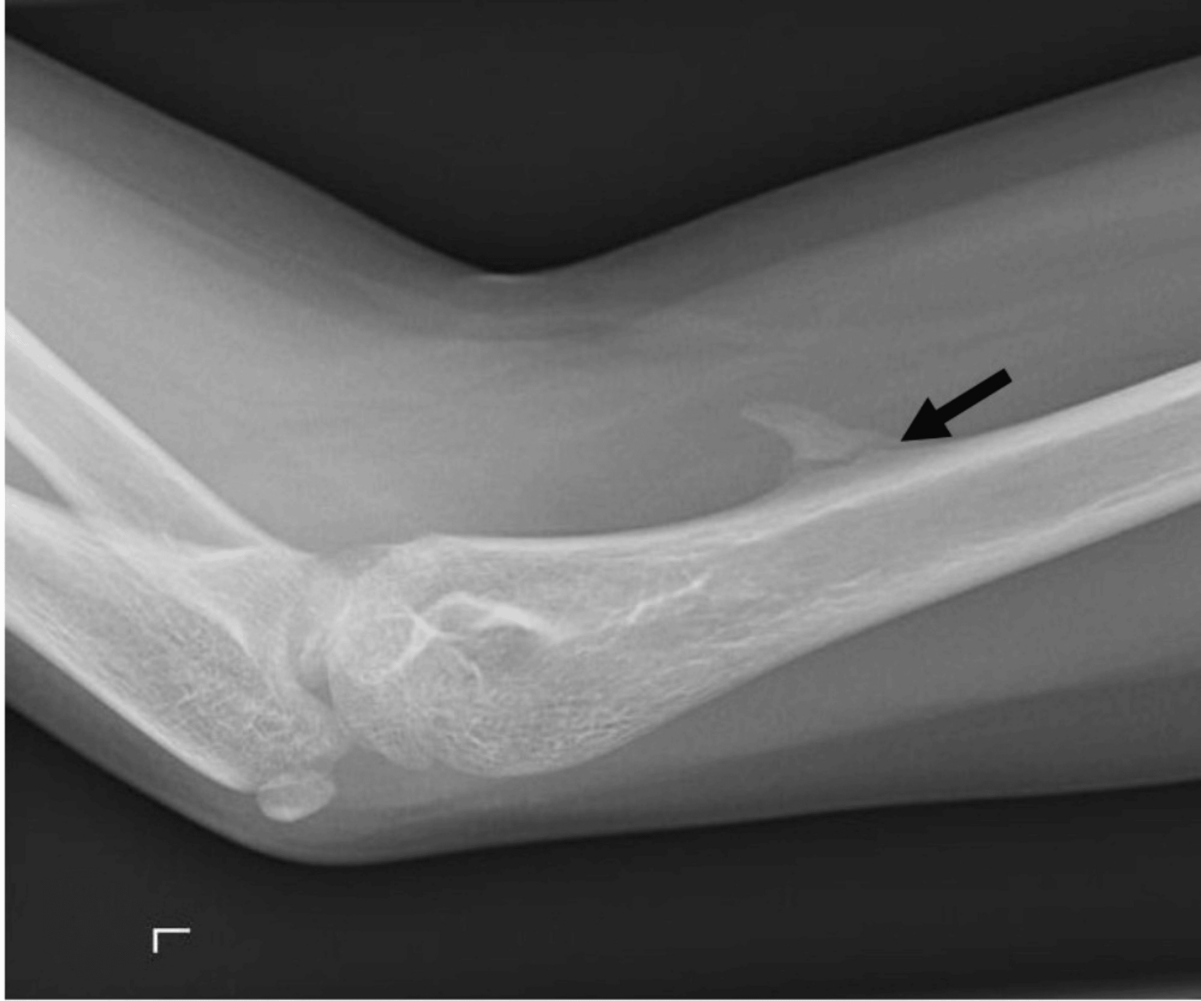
Oblique radiograph four weeks post-injury discloses fracture callus at the base of the lesion (arrow) consistent with healing fracture.
